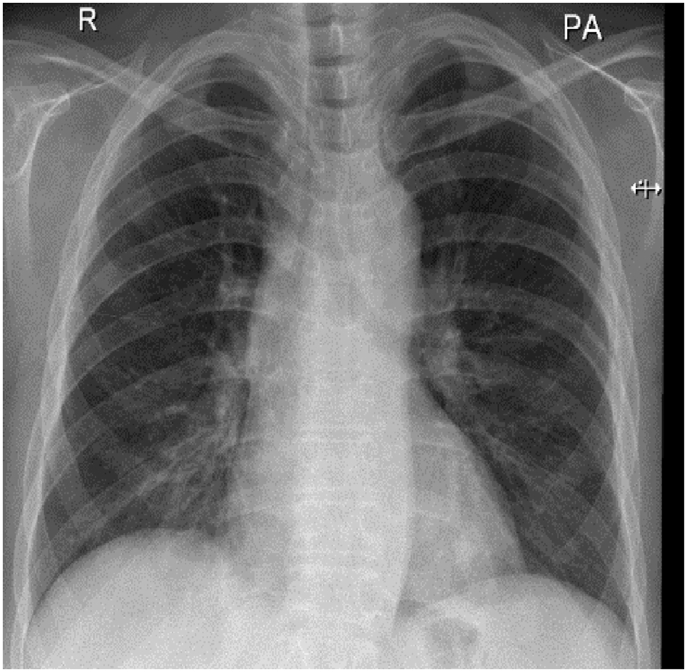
Chest x-ray, PA view, December 2020: normal chest x-ray.
